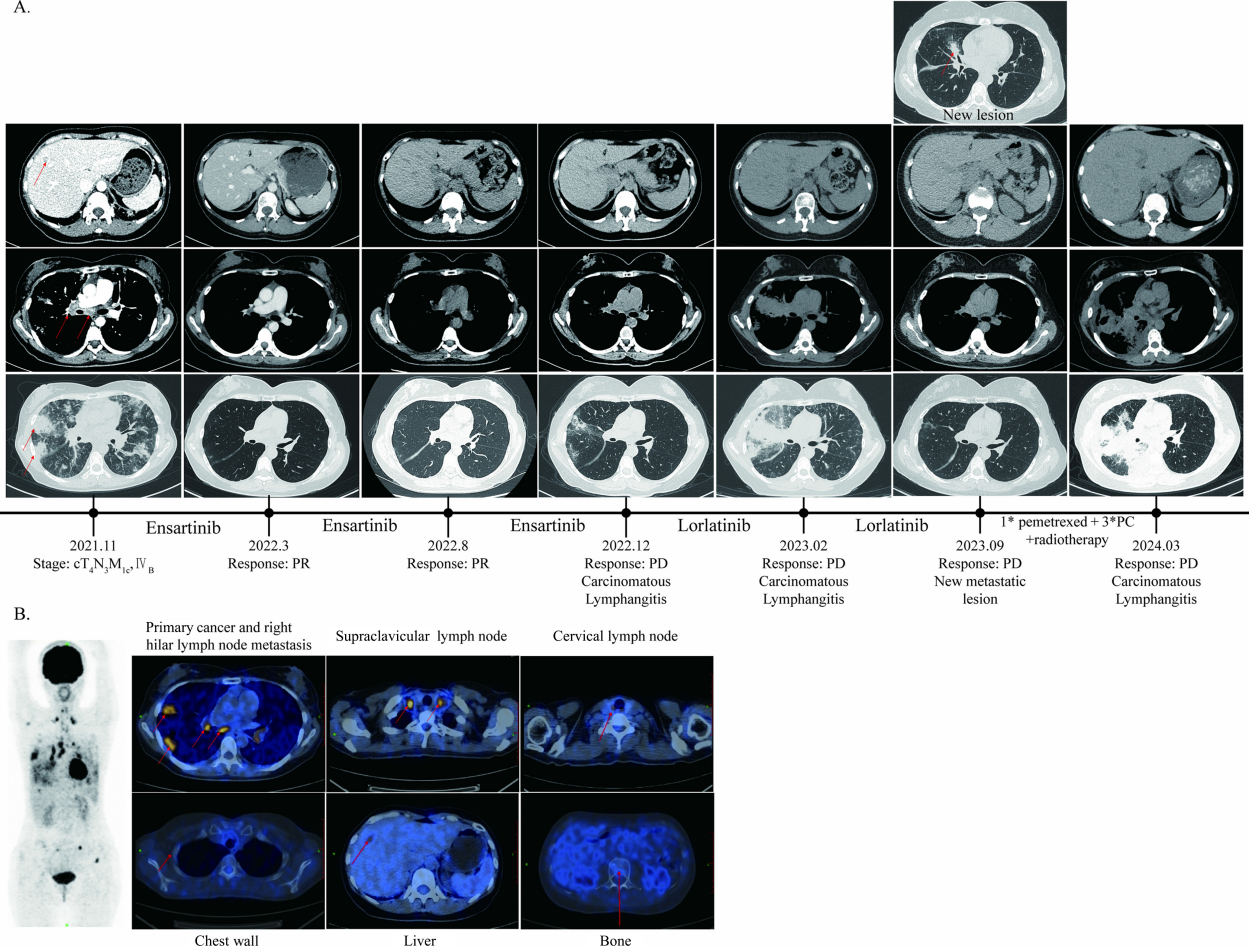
The initial PET/CT scans and CT scans before and after treatment of Case 2. (A) CT scans of Case 2 before and after treatment. Ensartinib: 225 mg orally per day; PC, pemetrexed + carboplatinum; PD, progressive disease; PR, partial remission. (B) Initial PET/CT scans of Case 2. The red arrows point to the primary lung cancer, metastatic lymph node, and metastatic lesions. Lorlatinib: 100 mg orally per day.
